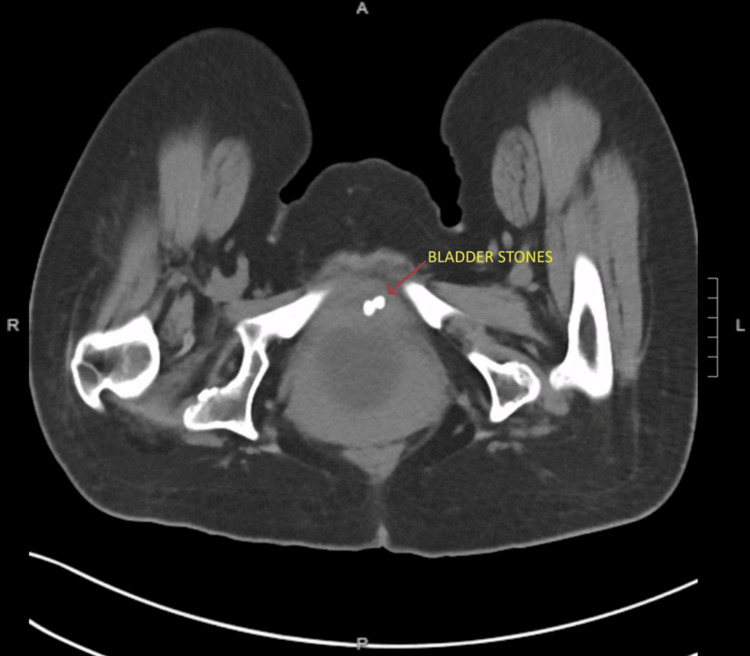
Cross-sectional image of the patient's preoperative CT scan showing two large, non-obstructing bladder stones.
